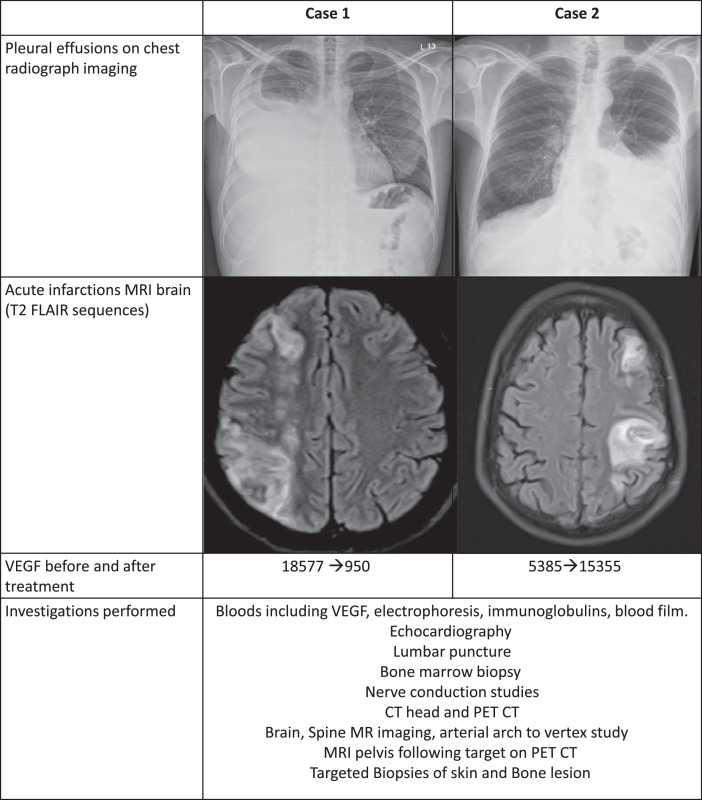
Investigations for each case Description: table describing investigations performed and key results including chest radiographs and MRI images.
